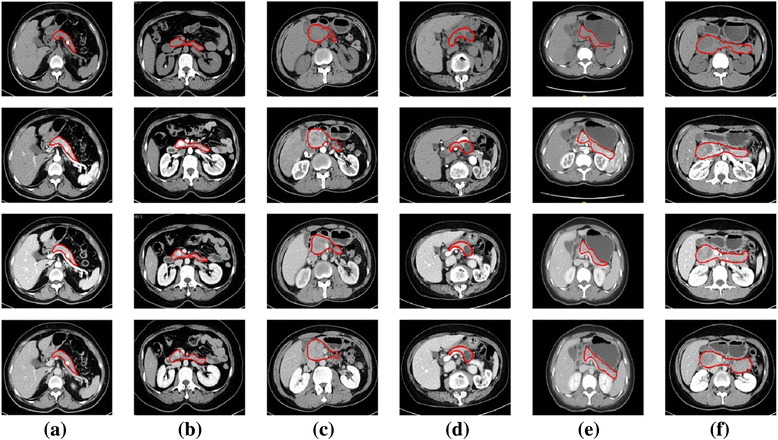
Examples of the six types (including non-disease) of abdomen CT image for (a) Healthy, (b) PNET, (c) PDAC, (d) IPMN, (e) SCA, (f) SPT. From row1 to row4 are non-enhanced phase, arterial phase, portal phase, delayed phase
